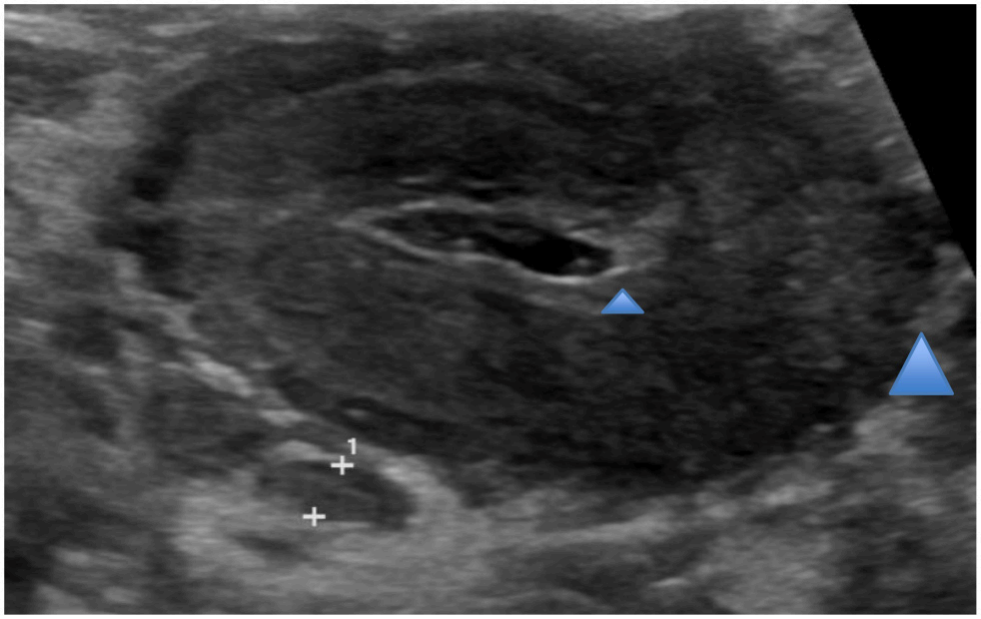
Abdominal ultrasound. Marked thickening of colon loops within mid epigastric and left upper quadrant representing transverse (see blue arrows) and descending colon.
